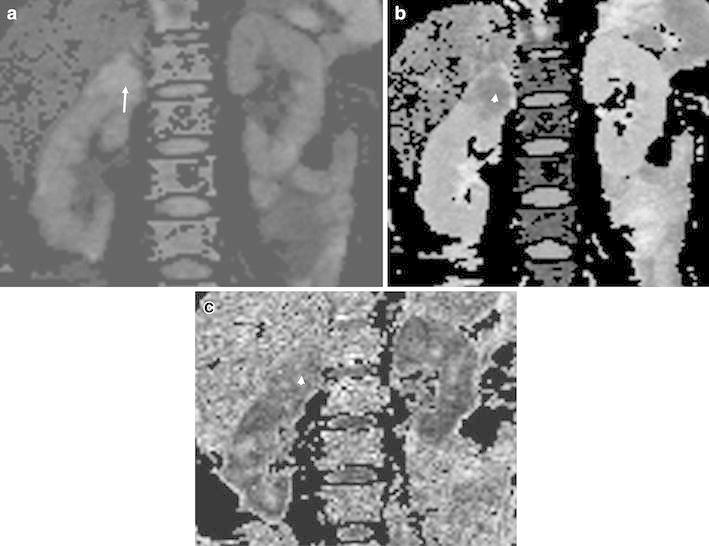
a–c. Images in 47-year-old woman with low-fat RAML. a DWI map RAML show even high signal (*arrow*). b Corresponding ADC map shows even low signal (*arrow head*). c FA map shows intermediate signal (*arrow head*)
